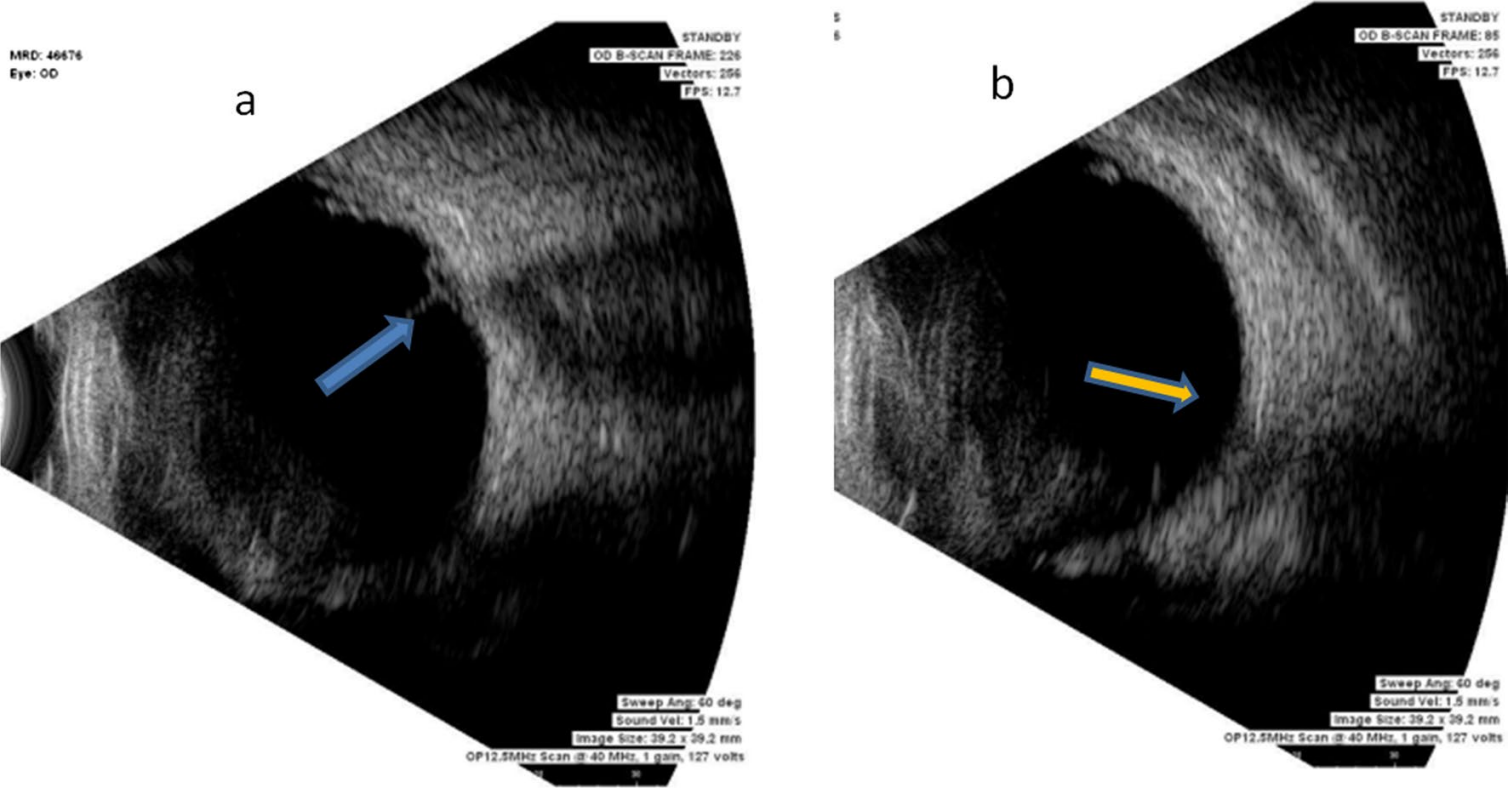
Preoperative ultrasound scans (a) of the patient demonstrates obvious traction adjacent to the disc (blue arrow). Ultrasound B scan at the 5-year follow-up shows that the traction remains relieved in the area adjacent to the disc (b). The patient’s vision recovered to 6/12 and has been maintained over 5 years. In the Ultrasound, the blue arrow points to the tractional detachment preoperatively and the yellow arrow points to the released traction postoperatively
